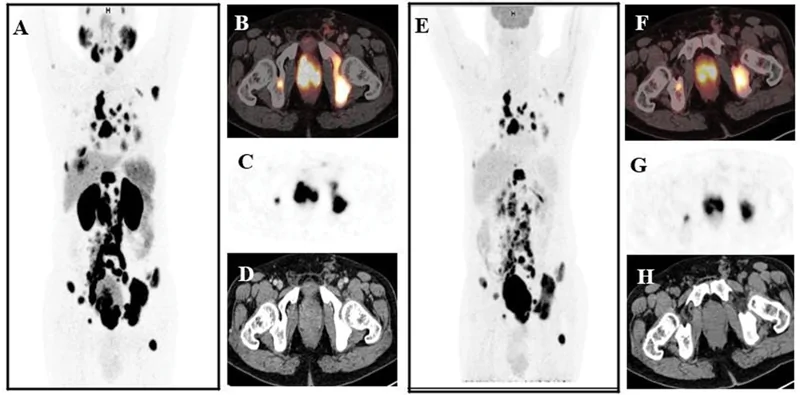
Concordance between gallium-68 (Ga-68) prostate-specific membrane antigen (PSMA) positron emission tomography/computed tomography (PET/CT) and F-18 fluorodeoxyglucose (FDG) PET/CT. (
